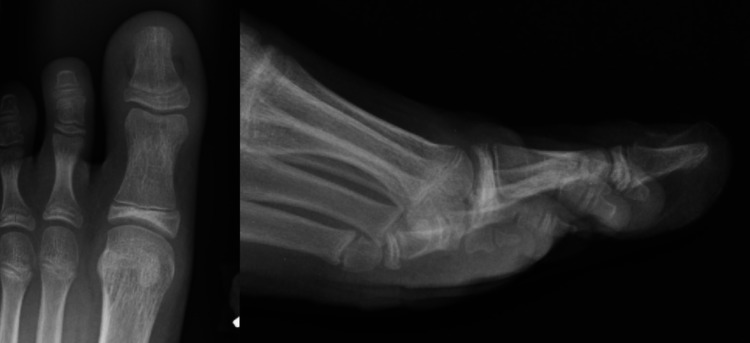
AP (left) and lateral (right) radiographs of the left great toe six weeks post-operatively demonstrating interval removal of the Kirschner-wire and maintenance of reduction with healing of the Salter-Harris I fracture of the distal phalanx. AP, anteroposterior.
